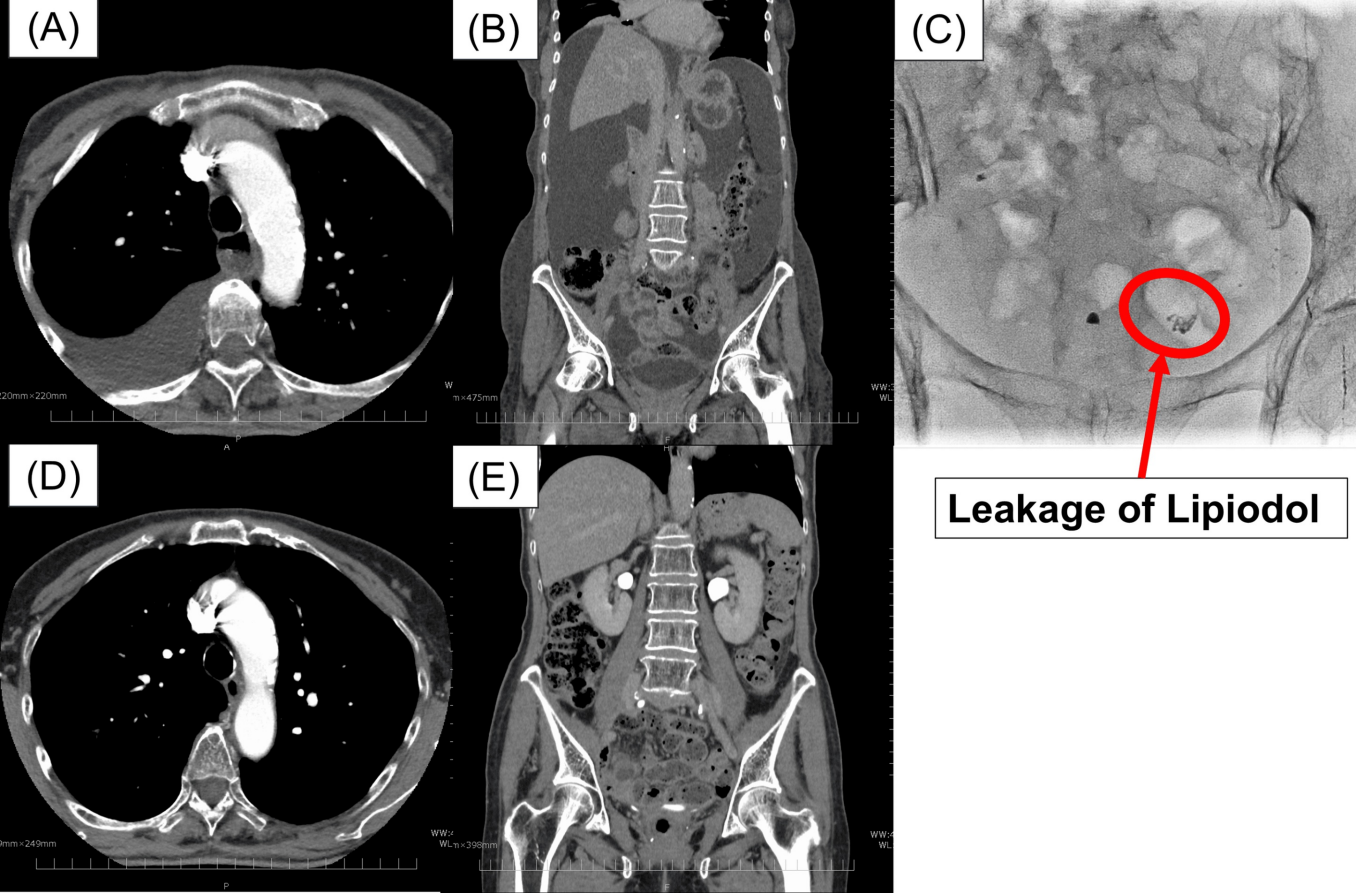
Imaging findings in this case (A) Chest CT at seven months postoperatively shows a right-sided pleural effusion without pleural nodules suggestive of malignant dissemination. (B) Abdominal CT at seven months postoperatively shows massive ascites but no peritoneal nodules suggestive of metastasis. (C) Lymphangiography demonstrates leakage of Lipiodol (red circle) into the abdominal cavity. (D) Chest CT at 18 months postoperatively shows resolution of the pleural effusion. (E) Abdominal CT at 18 months postoperatively shows no ascites.
